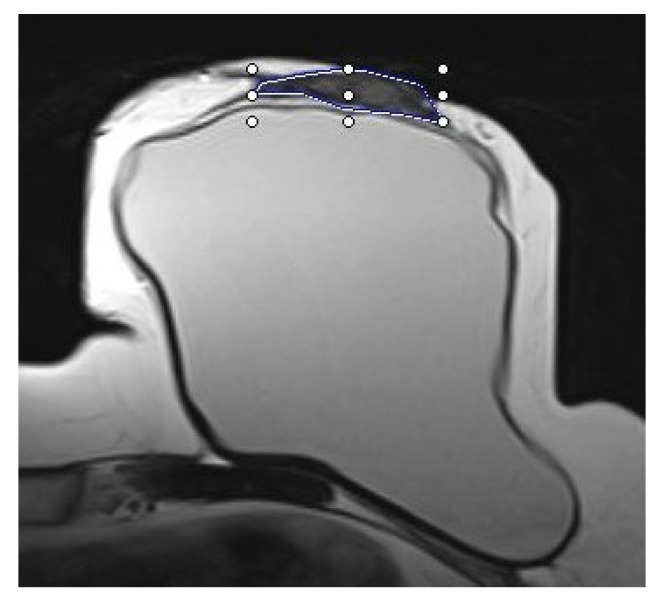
Volumetry of residual glandular tissue after risk-reducing mastectomy with implant T2-weighted image. The measurements were made using the image processing software Syngo.via (Siemens Healthcare GmbH, Erlangen, Germany).
